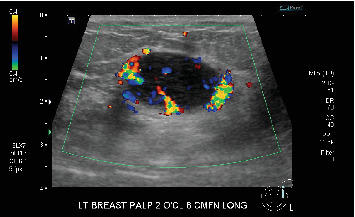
Targeted ultrasound of the left upper outer breast mass demonstrated a smoothly marginated hypoechoic mass 8 cm from the nipple and measuring 2.8 × 1.6 × 2.8 cm with internal and marked color Doppler vascular flow.
